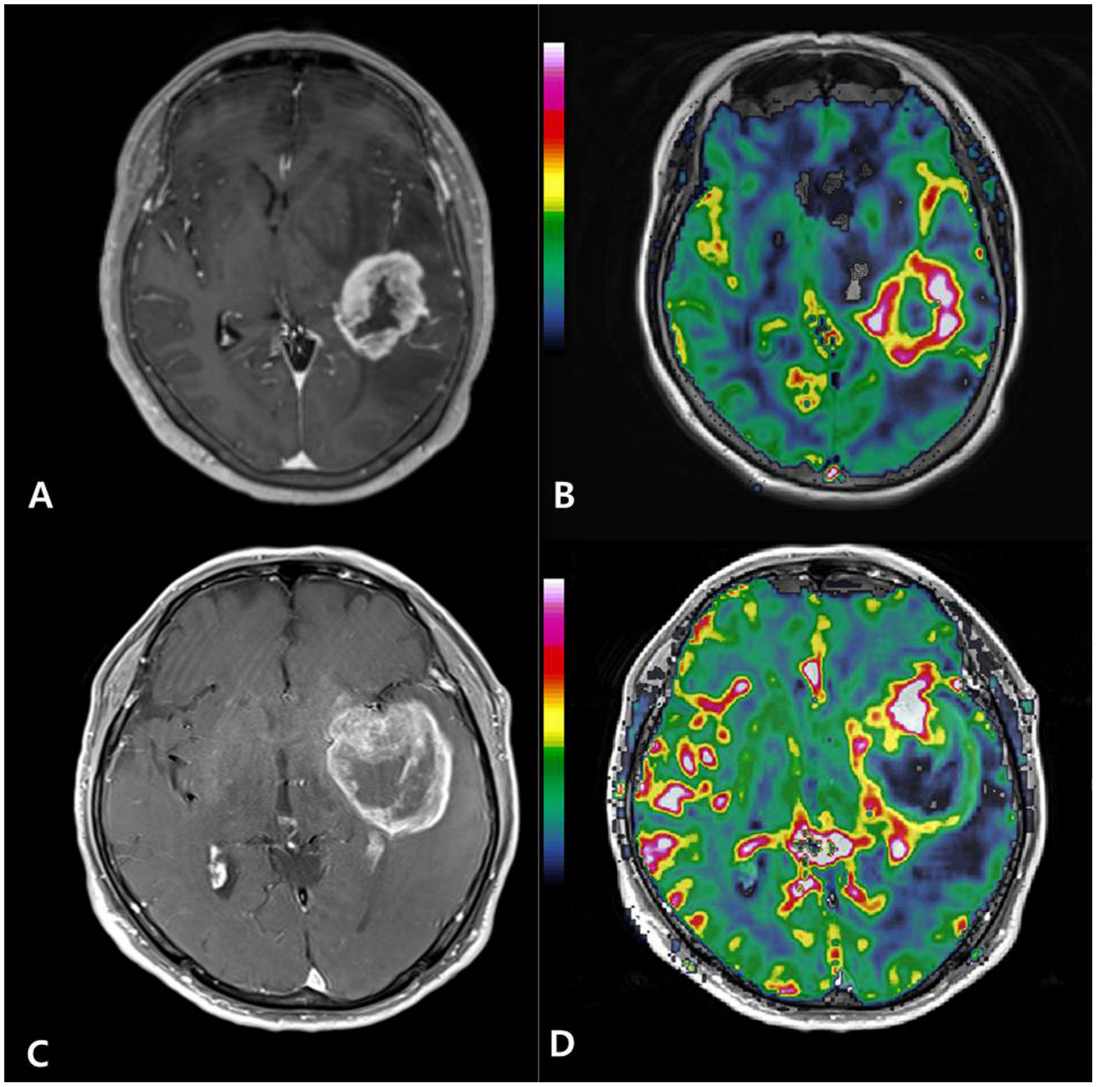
Glioblastomas with aggressive genetic profiles show high normalized relative tumor blood volume (nTBV). (A, B) A 66-year-old woman had glioblastoma with EGFR expression (3+), PTEN loss (+), MGMT methylation (−), and a Ki-67 index of 39%. The tumor showed high nTBV (27.5).(C, D) A 70-year-old man had glioblastoma with EGFR expression (3+), PTEN loss (+), MGMT methylation (−), and a Ki-67 index of 27%. The tumor showed high nTBV (12.84). (A, C) Contrast-enhanced T1-weighted axial image, (B, D) normalized relative cerebral blood volume (nCBV) map overlaid on structural contrast-enhanced T1-weighted axial image.
